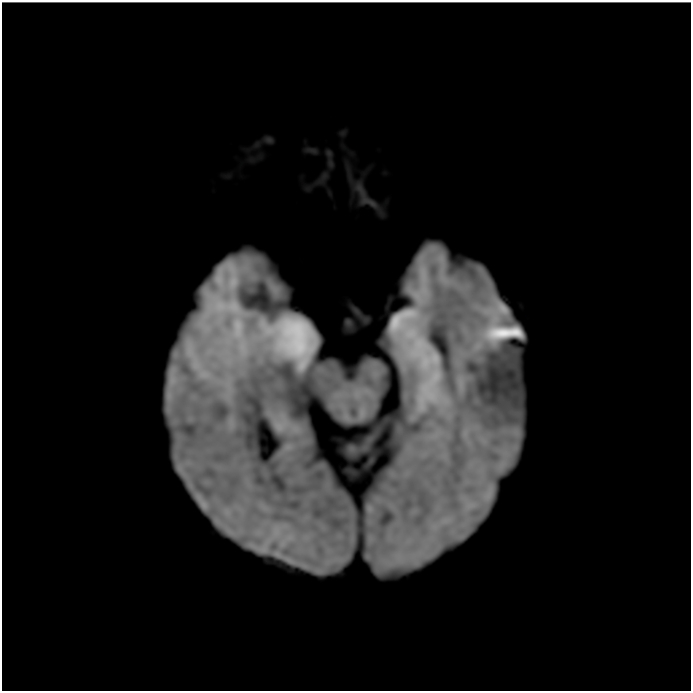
MRI-DWI of patient 5; showing restricted diffusion in right > left mesial temporal lobes. DWI: Diffusion-Weighted Imaging.
